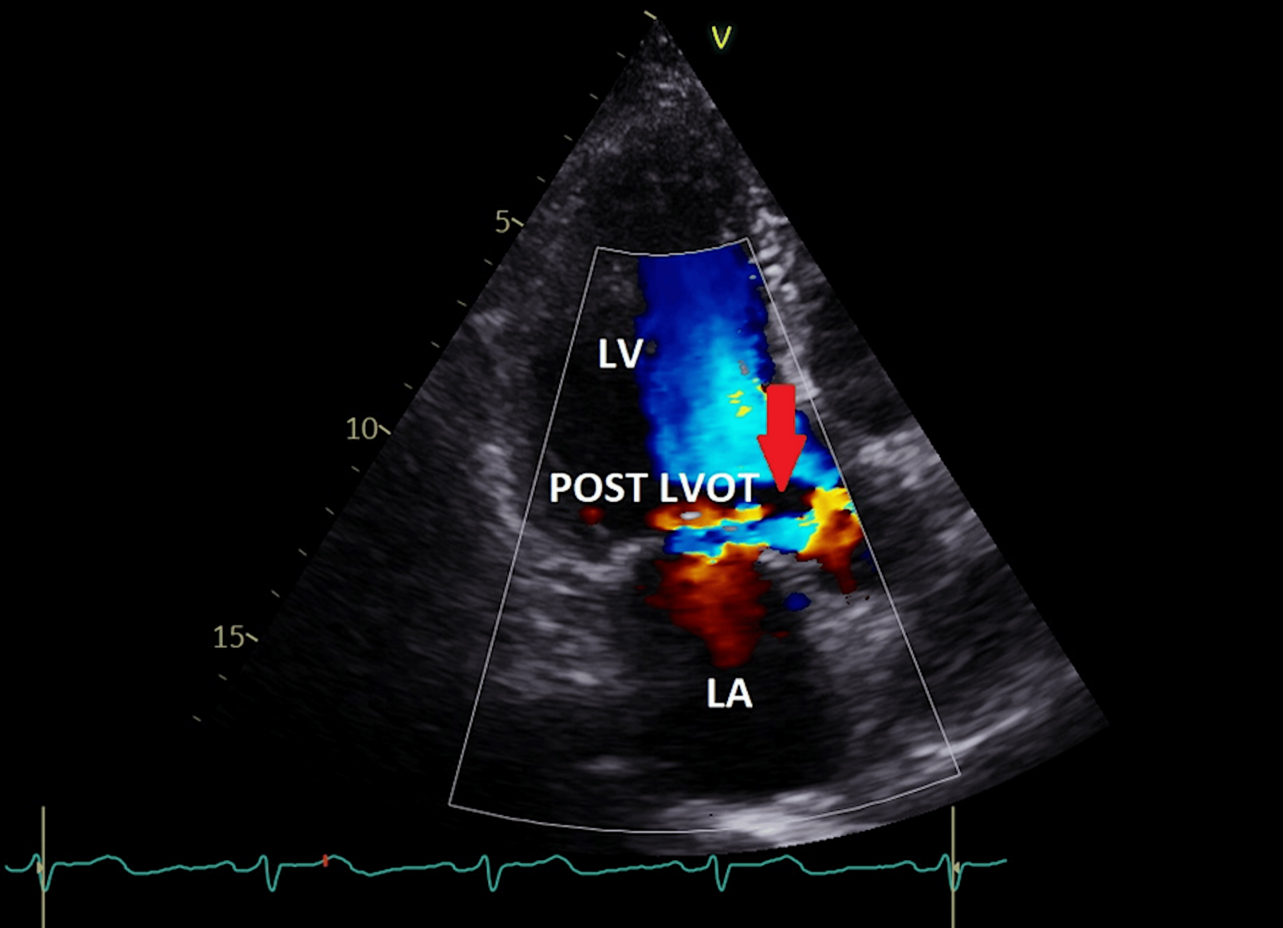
Apical three-chamber view showing color flow aliasing in the posterior part of the LVOT (red arrow) with normal color flow in the anterior part of the LVOT. This color aliasing in the LVOT marks the onset of the Gerbode defect from the left ventricle to the right atrium. LA = left atrium; LV = left ventricle; POST LVOT = posterior left ventricular outflow tract
